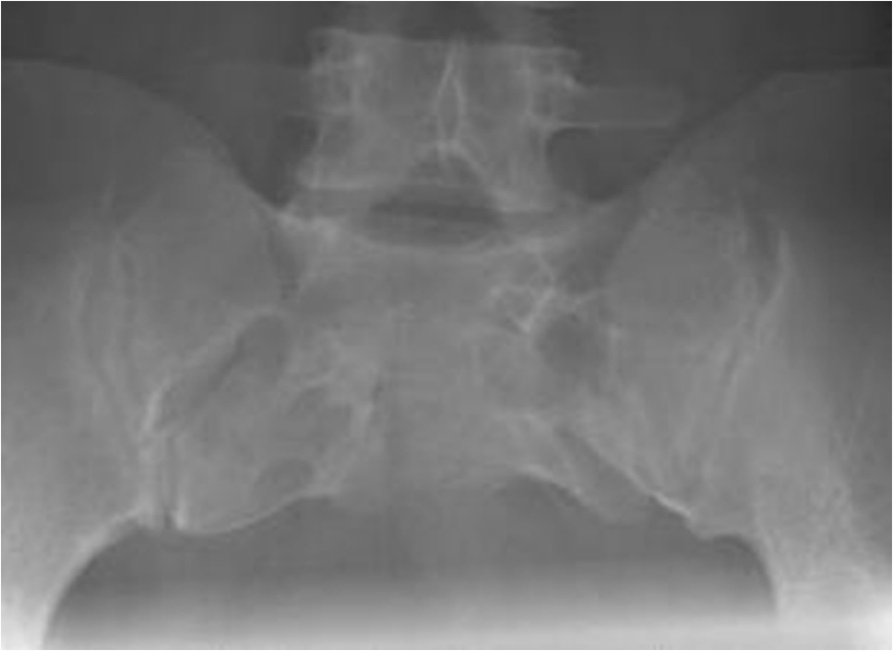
On conventional sacral X-ray a dysgenetic sacrum was seen.
